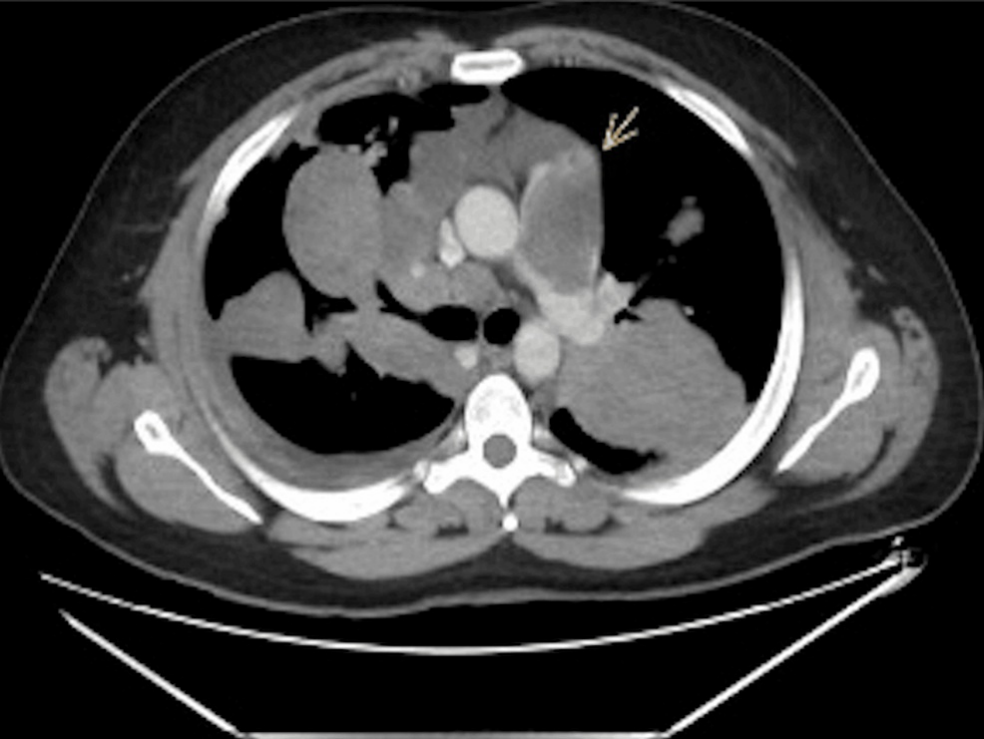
Axial CT chest with IV contrast study showed main pulmonary artery invasion by a soft tissue mass. CT-guided biopsy was taken from the left lung lesion. Computed tomography (CT); Intravenous (IV)
